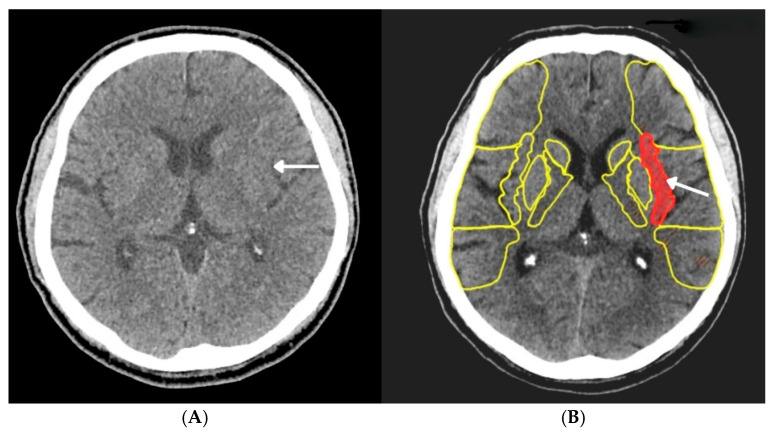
Computed tomography of the head revealing a discreet blurring of the left insular band (A) and left insular ribbon involvement (area marked in red) according to Alberta stroke program early CT score (ASPECTS) (B) (marked by arrow).
